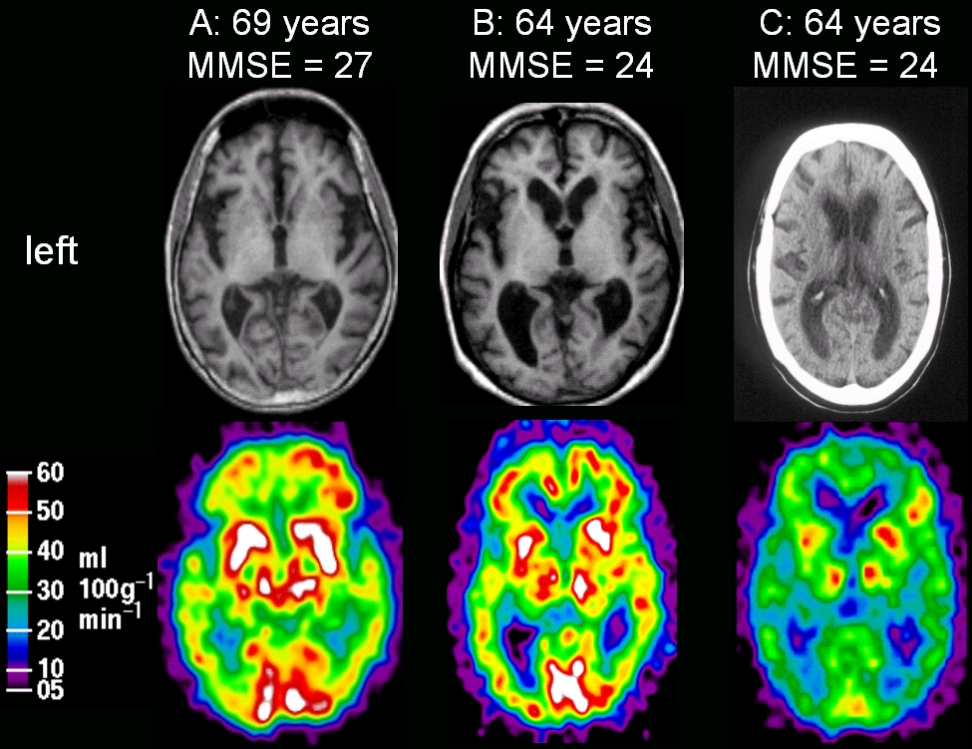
Representative brain scans in FTD-3 patients. Upper row: structural scans in FTD-3 with MRI for patients A and B, and a CT-scan for patient C. Lower row shows cerebral blood flow as measured by with H_2_^15^O -PET scanning for the same 3 patients.
